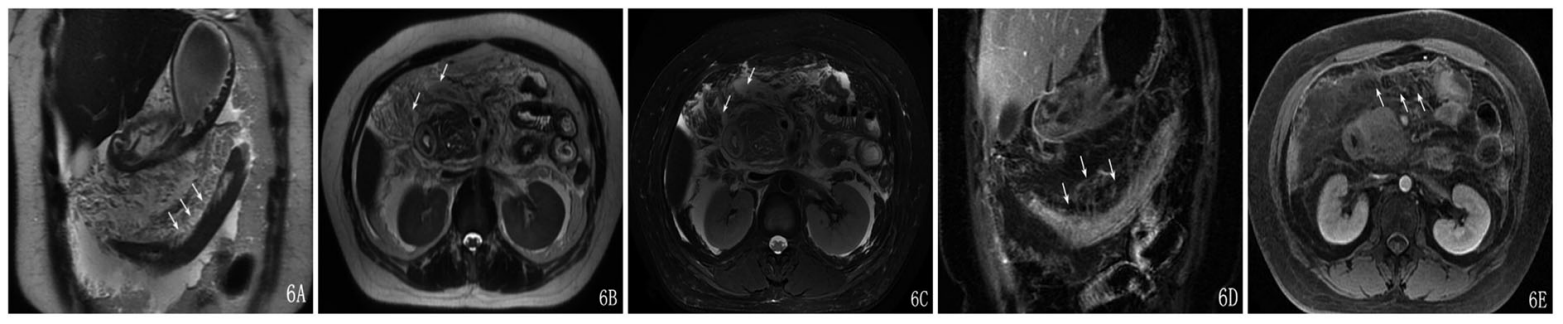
A 47-year-old female with severe AP and TMI was Grade III. The transverse mesocolon showed edema and thickening on a coronal SSFSE T2-weighted image (A). The transverse mesocolon showed effusions on an axial SSFSE T2-weighted image (B) and on an axial SSFSE T2-weighted image with fat suppression (C). The transverse-mesocolon-vessel involvement could be seen on the enhancement of the coronal image (D) and axial image (E).
